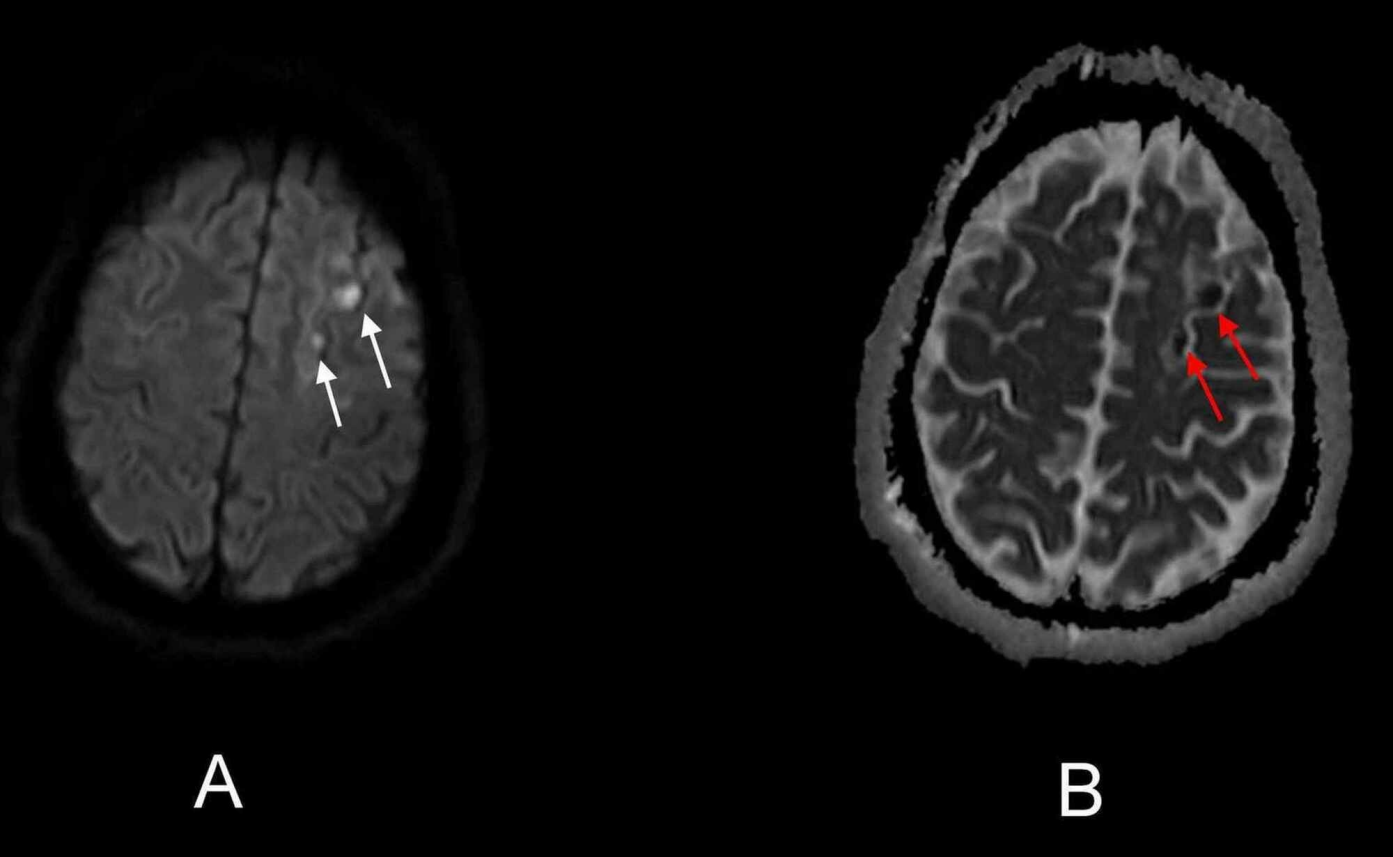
Axial DW and ADC sequence of the second brain MRI demonstrating new acute ischemic infarcts White arrows on axial DW sequence (A) and red arrows on axial ADC sequence (B) demonstrate two new acute ischemic infarcts in the left cerebral hemisphere manifested clinically as acute onset motor aphasia. DW - diffusion-weighted; ADC - apparent-diffusion-coefficient
